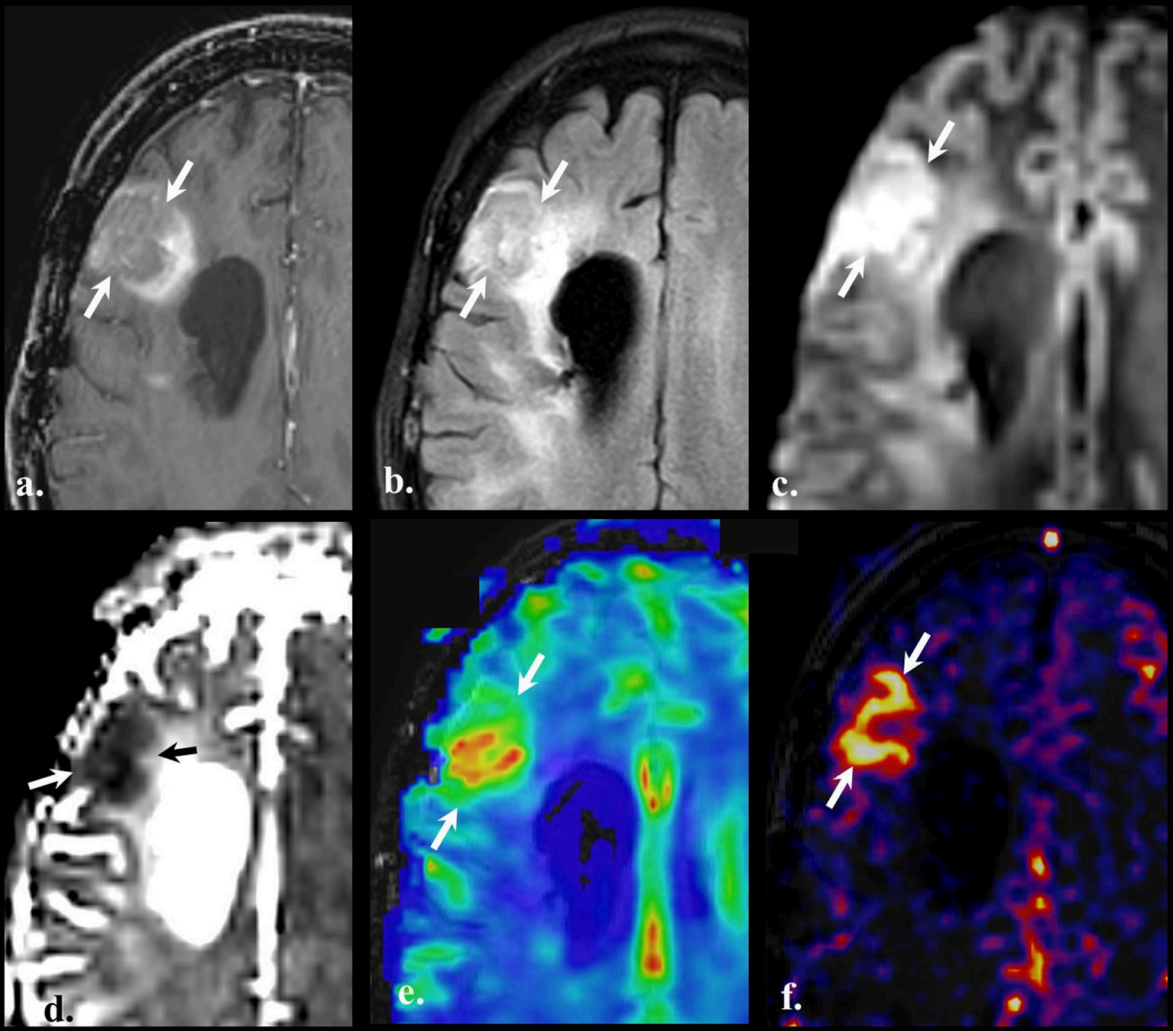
Perfusion-weighted imaging (PWI). Brain MRI of a old with a diagnosis of recurrent GBM (IDH wild-type, MGMT status unknown) on bevacizumab treatment. Axial contrast-enhanced T1w (a), FLAIR (b), DWI (c), ADC (d), Dynamic susceptibility contrast (DSC) (e), and Pseudo-continuous arterial spin labeling (f). Compared with the baseline scan, an evolving non-enhancing FLAIR hyperintense lesion (arrows on a and b) is noted on the right frontal lobe in the vicinity of the surgical cavity (not shown). The lesion shows high signal on DWI (arrows on c) with the reciprocal dark appearance on ADC map (arrows on d) due to hypercellularity. Dynamic susceptibility contrast depicts increased cerebral blood volume (CBV) (arrows on panel e), and pseudo-continuous arterial spin labeling (pCASL) reveals an increment in the cerebral blood flow in the same location. PWI findings are indicative of vascular proliferation.
